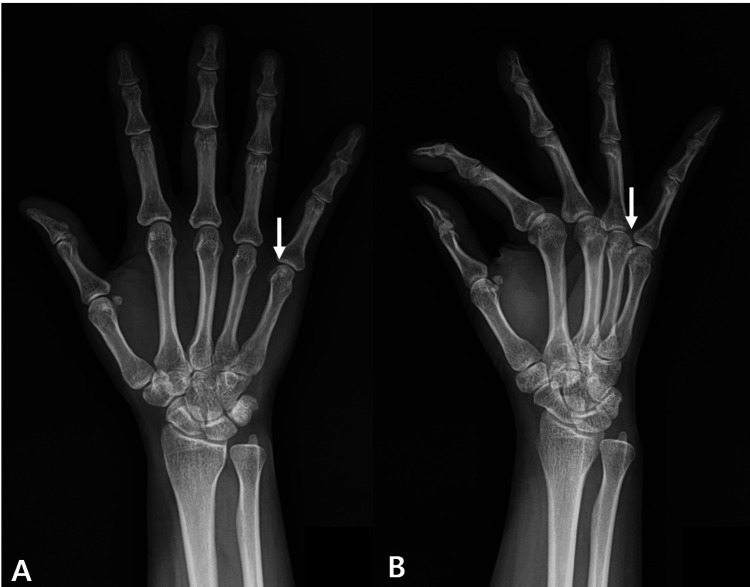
Calcifications adjacent to the sesamoid bone can be seen on X-ray. Calcifications distinct from the sesamoid are observed in the (A) AP view (white arrow) and (B) oblique view (white arrow).
